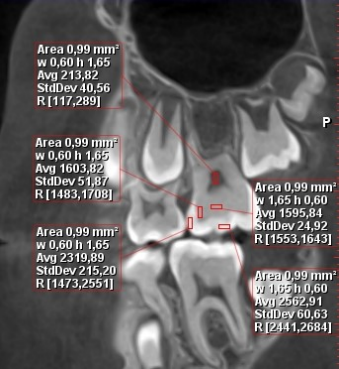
Measuring the radiodensity (in Hounsfield units) of a first permanent molar of a boy (10 years old) under cone-beam computed tomography. H: height; W: width.
